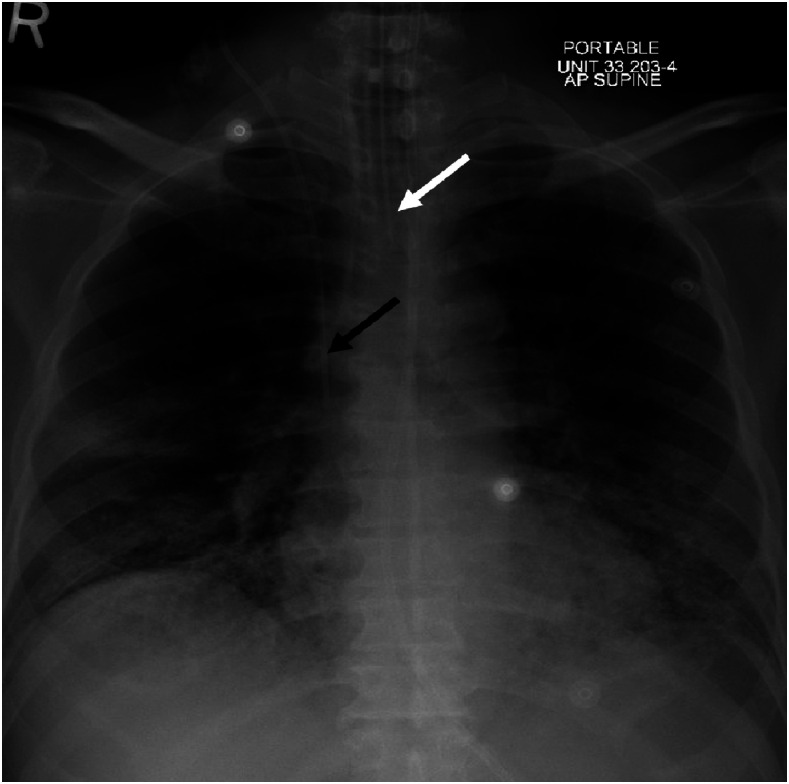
Chest X-ray examination indicating the endotracheal tube (white arrow) and central line insertion (black arrow) with no complications. Repeat demonstration of bilateral infiltrates.
